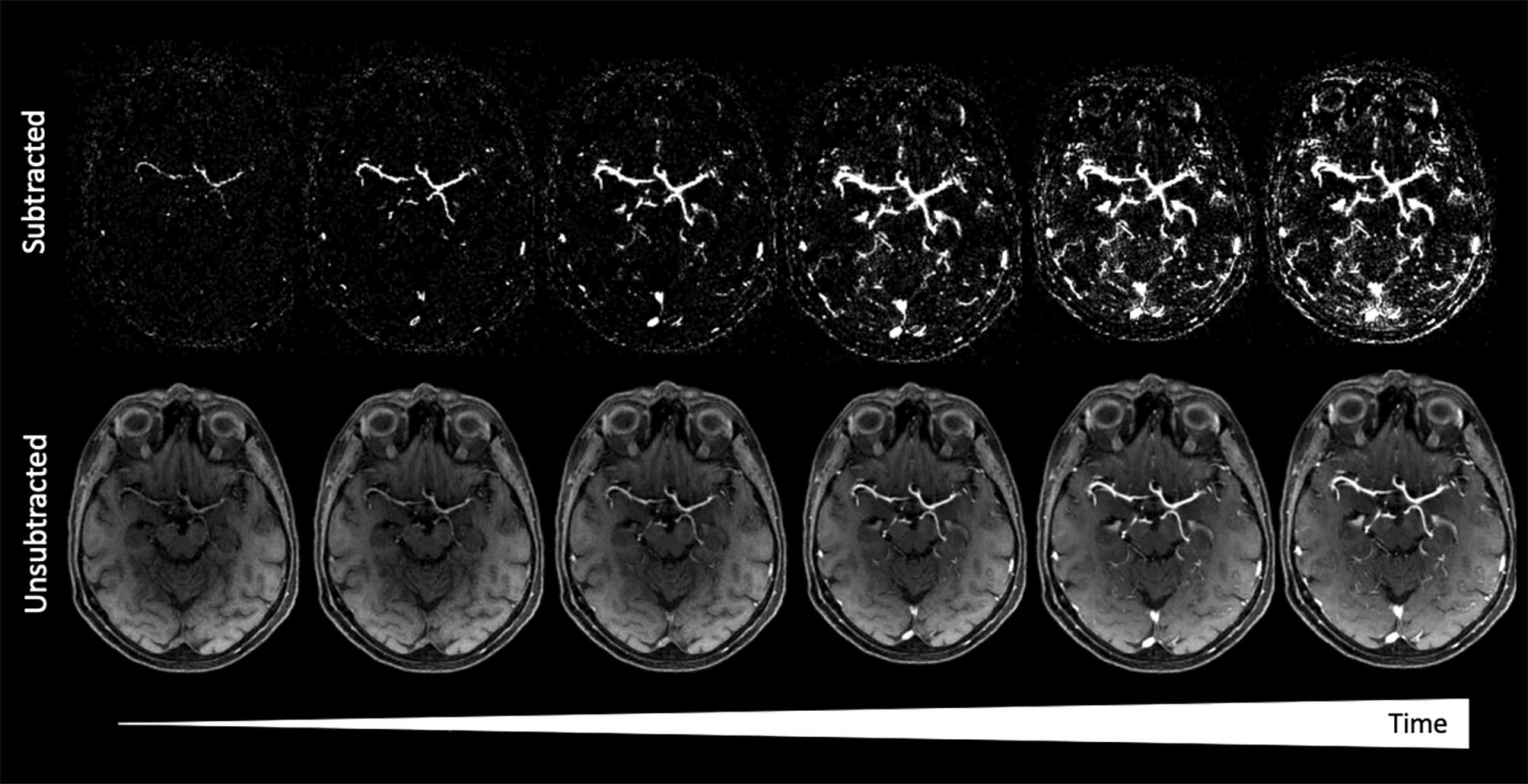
GRASP MRI from a 63-year-old woman imaged for headache reconstructed as time-resolved MRA images. Paired subtracted (top row) and source (bottom row) axial slices obtained from the 3D GRASP imaging volume at the level of the Sylvia cistern and fissure. Dynamic structural images reconstructed from 5 spokes of a golden-angle radial acquisition are shown, demonstrating the earliest arterial through the venous phases of gadolinium bolus passage.
